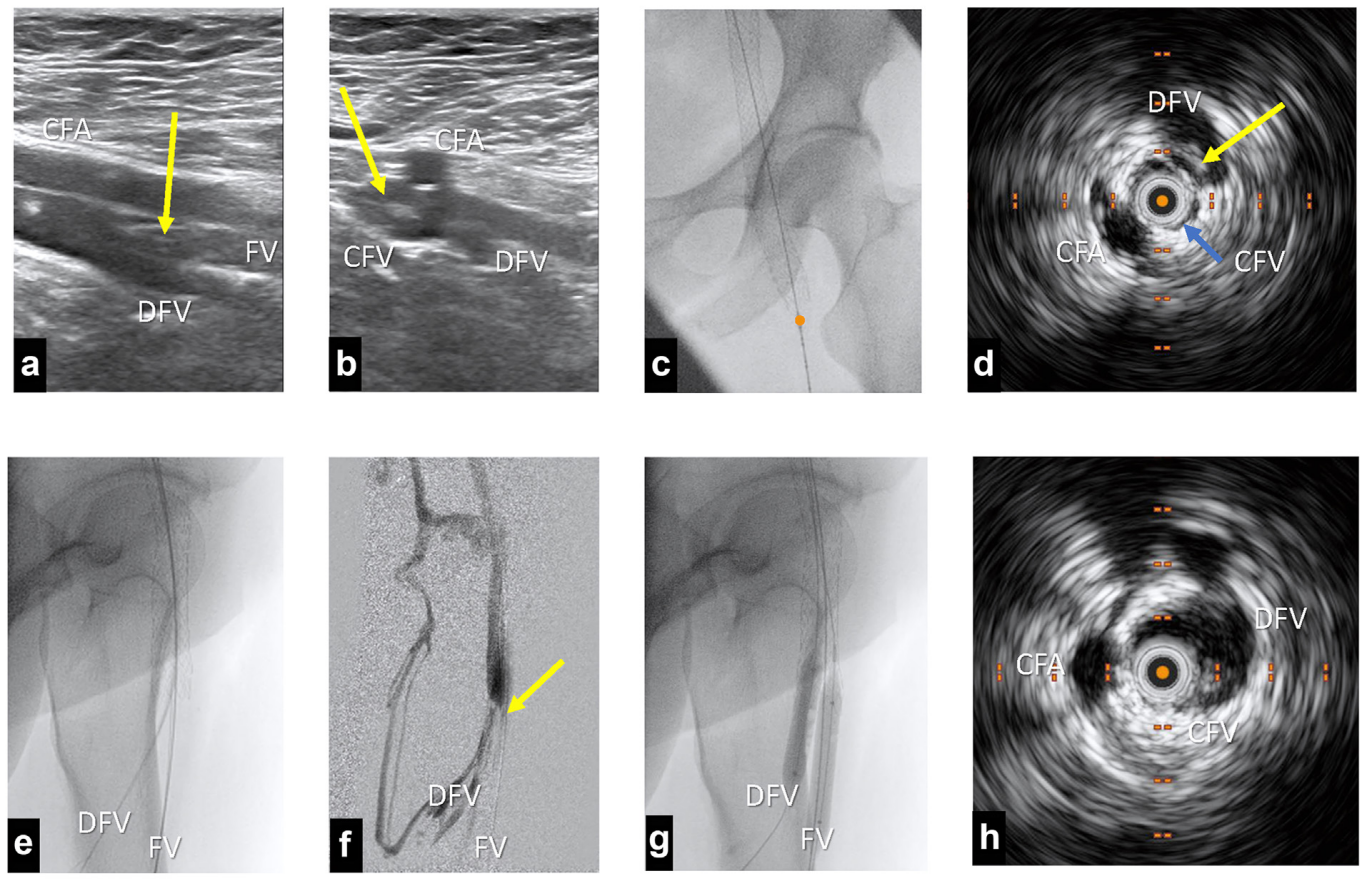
An example showing the role of preoperative DUS and intraoperative IVUS in checking the extension of post-thrombotic changes and how to ensure the single inflow vein can drain the blood into the stents. (A) Longitudinal view of the preoperative DUS showing the post-thrombotic involvement (*yellow arrow*) of CFV and FV which leaves the DFV as the single inflow vein. (B) Cross-sectional view of the preoperative DUS in same case ensures open and free DFV. Yellow arrow shows the post-thrombotic occluded CFV. (C) AP intraoperative fluoroscopy after stent implantation with simultaneous IVUS. The tip of the IVUS catheter (*orange dot*) at distal landing zone checking the DFV. (D) Acquired image of IVUS in the same position as (C) showing that the post-thrombotic synechiae (*yellow arrow*) blocks the orifice of DFV and hinders the inflow. (E) Lateral fluoroscopy image shows the (transjugular) retrograde cannulation of DFV. (F) Phlebography shows the post-thrombotic synechiae (*yellow arrow*) blocks the flow into the stents with dominated flow into the collaterals. (G) Simultaneous ballooning the FV (antegrade) and DFV (retrograde) to rupture the synechiae. (H) IVUS image after ballooning the DF and DFV showing the free pass way from DFV into CFV. *AP*, anteroposterior; *CFV*, common femoral vein; *DFV*, deep femoral vein; *DUS*, duplex ultrasound; *FV*, femoral vein; *IVUS*, intravascular ultrasound.
